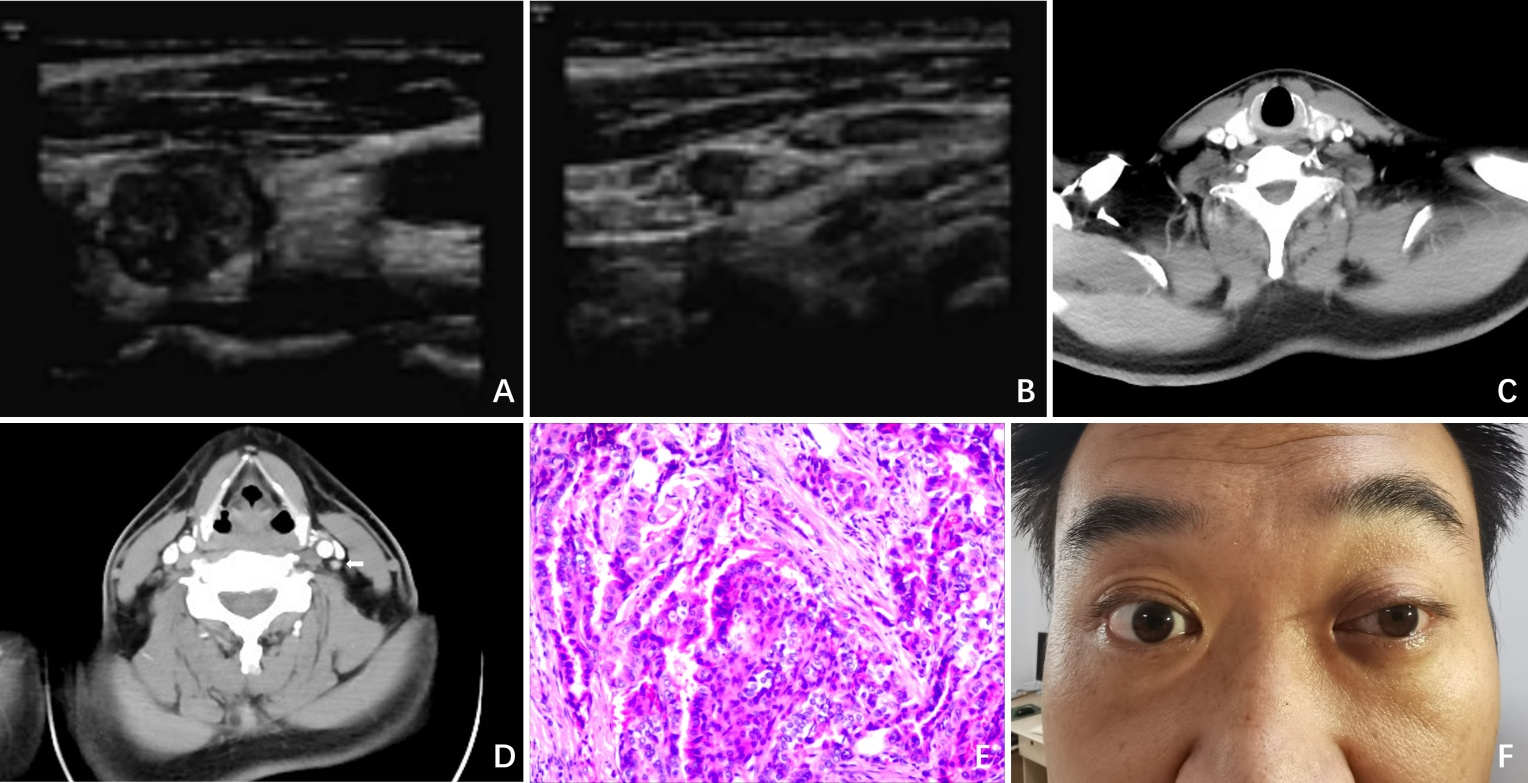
Clinical data. (A) Ultrasound revealed a nodule situated in the left lobe of the thyroid, measuring 1.4cm x 1.4cm x 1.6cm, with low echogenicity and containing strong echo spots; (B) Ultrasound revealed a 0.6cm lymph node containing strong echo spots in the vicinity of the left carotid artery sheath; (C) Enhanced CT revealed a significantly enhanced nodule in the left lobe of the thyroid; (D) Enhanced CT revealed a markedly enhanced lymph node at level II of the left neck (white arrow); (E) Postoperative pathology indicated PTC; (F) On POD 3, ptosis of the left eyelid, miosis, and anhidrosis on the ipsilateral face was observed in the patient.
